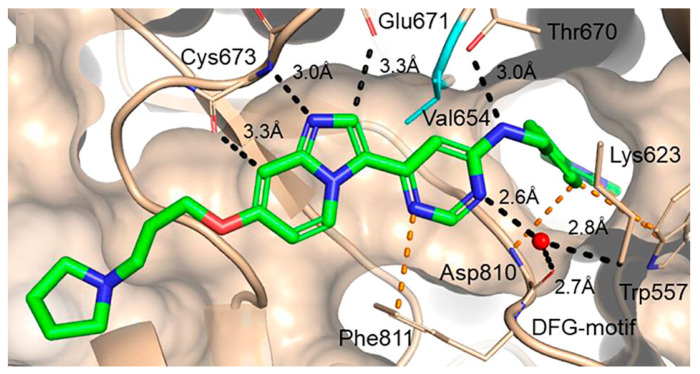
X-ray structure of 135j (2.1 A resolution, PDB-ID: 7ZW8) in complex with the kinase domain of c-KIT. Reprinted with permission from Ref. [51].
